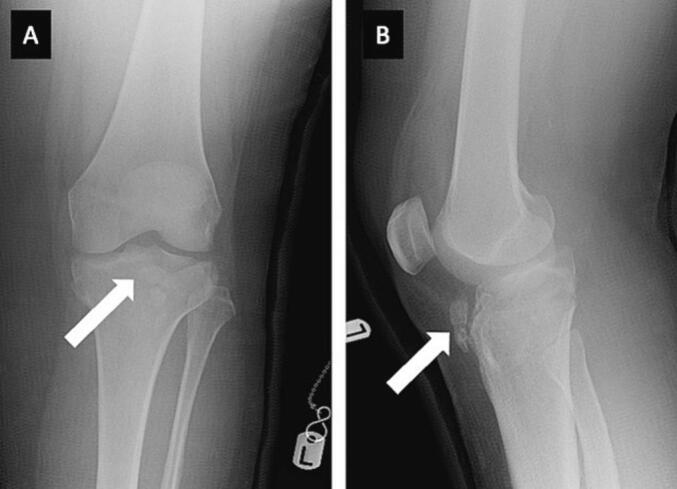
Anteroposterior (A) and lateral (B) radiographs of the left knee joint, obtained at injury presentation. These X-ray images demonstrated a hyperextension-bicondylar communited injury of the left tibial plateau (white arrows).
